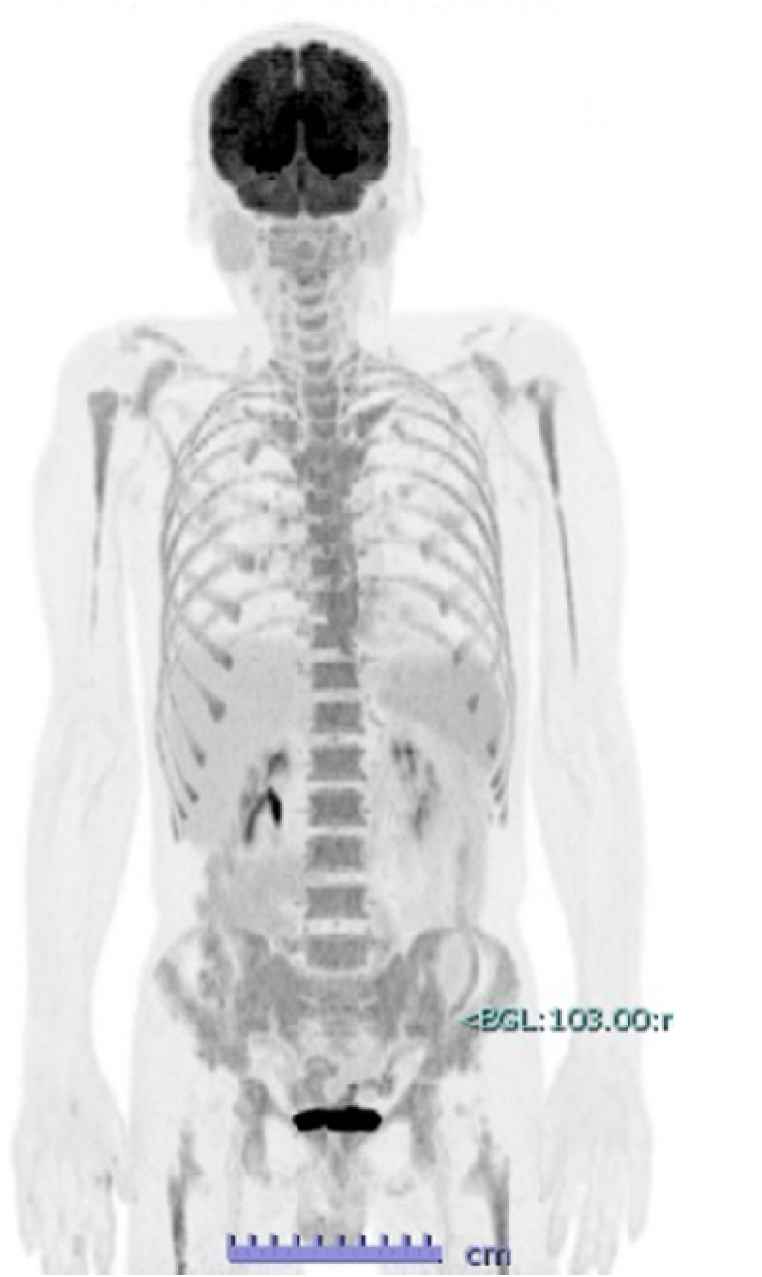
PET-CT performed two months prior to admission. No abnormal FDG uptake was observed in the primary lesion or other organs.
